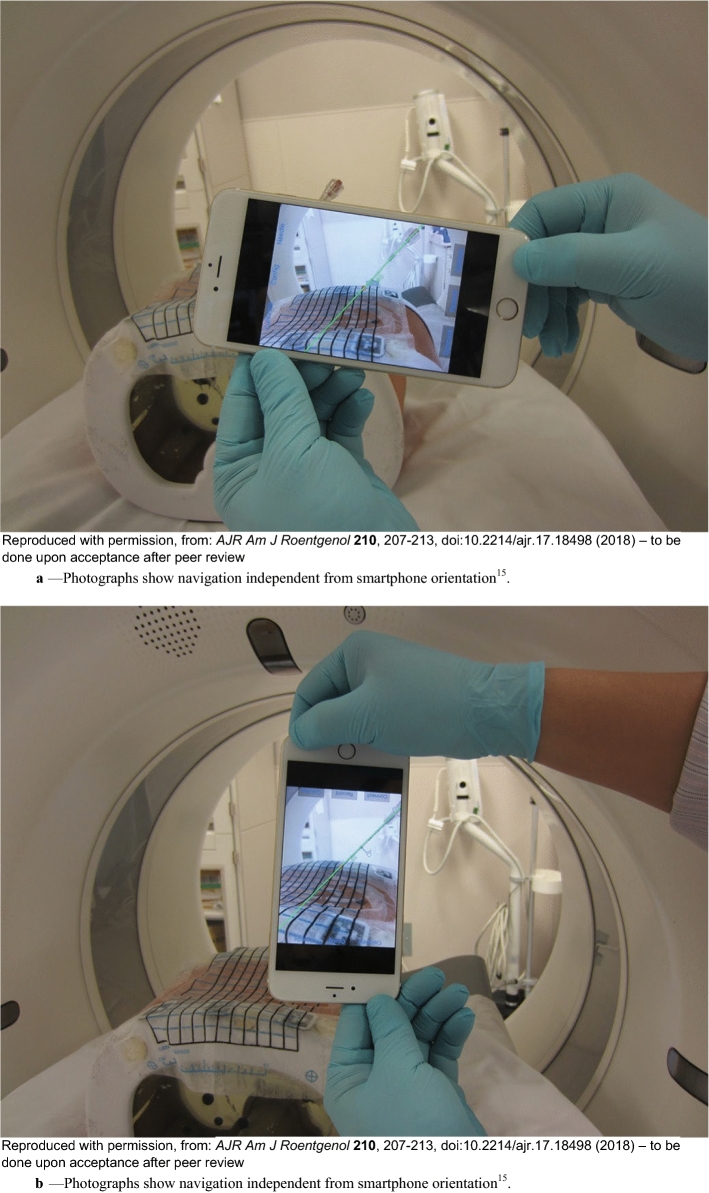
a Photographs show navigation independent from smartphone orientation [15]. a Photographs obtained with user holding smartphone in landscape A and portrait B orientations show that path angle (*green line*) displayed on smartphone is always referenced to CT. b Photographs show navigation independent from smartphone orientation [15]. b Photographs obtained with user holding smartphone in landscape A and portrait B orientations show that path angle (*green line*) displayed on smartphone is always referenced to CT
